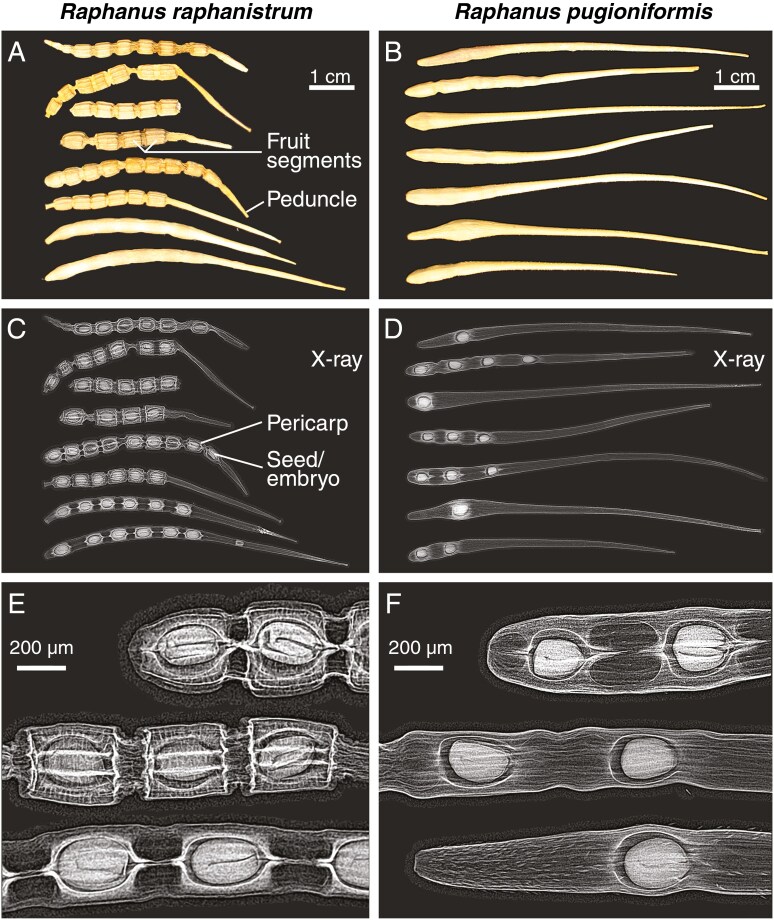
Fruit and seed morphology of wild radishes. (A) Mature fruits of *R. raphanistrum*; note that *R. raphanistrum* fruits may break apart into fruit segments. (B) Mature fruits of *R. pugioniformis*. (C) X-ray image of RR fruits shown in panel (A). (D) X-ray image of RP fruits shown in panel (B). (E) Enlargement of parts of panel (C) showing the internal morphology of *R. raphanistrum* fruit segments with seeds enclosed by pericarp (fruit wall) in seed chambers of individual fruit segments. (F) Enlargement of parts of panel (D) showing the internal morphology of *R. pugioniformis* fruits with seeds in seed chambers enclosed by pericarp (fruit wall). Note that the mature pericarp is dead tissue and that the X-ray imaging reveals distinct pericarp layers.
